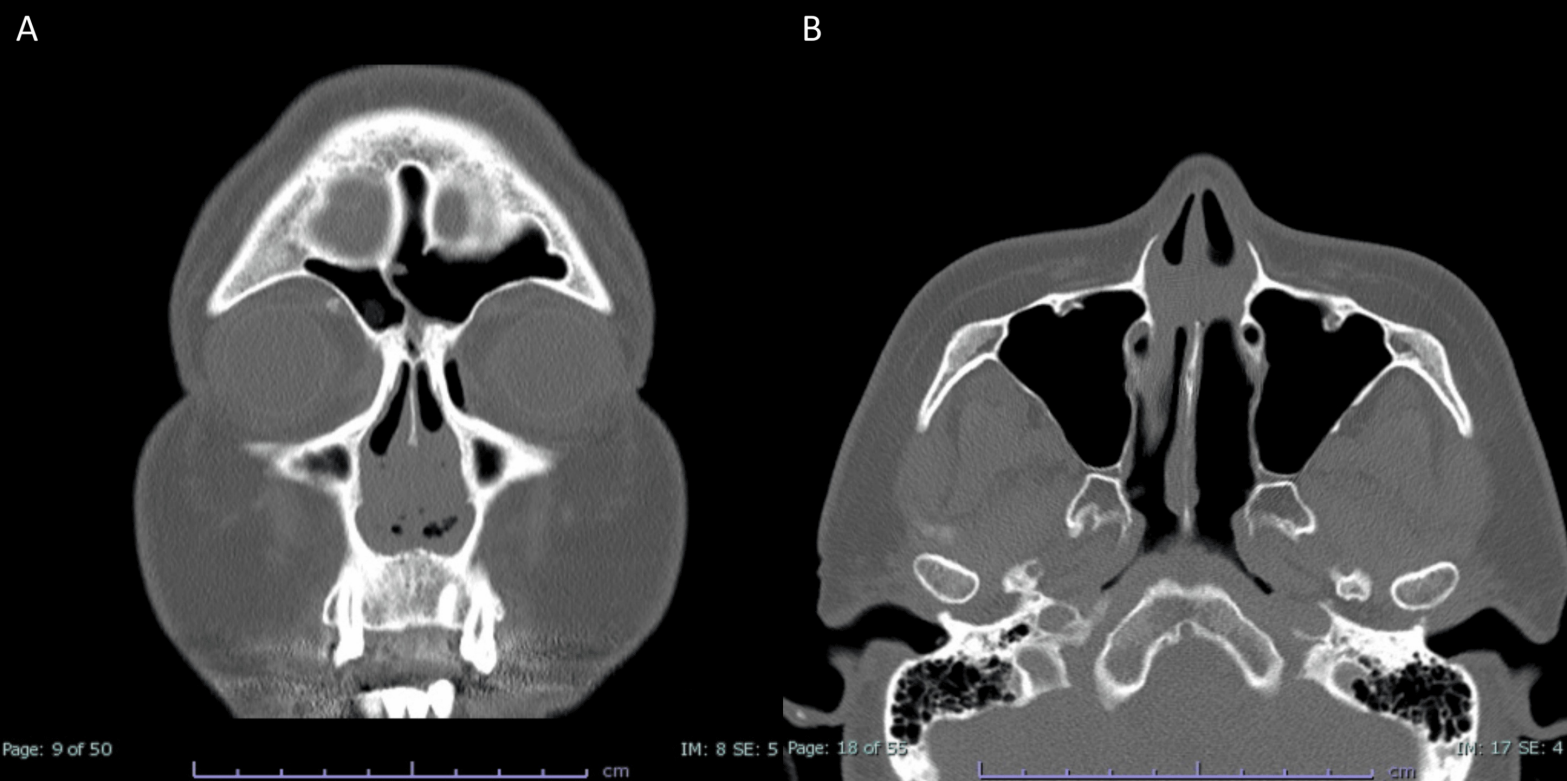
CT scan of the nasal cavity at the initial visit. A) Coronal view showing bilateral nostril obstruction due to adhesions. B) Axial image indicating that the adhesions are confined to the anterior part of the nasal cavity.
